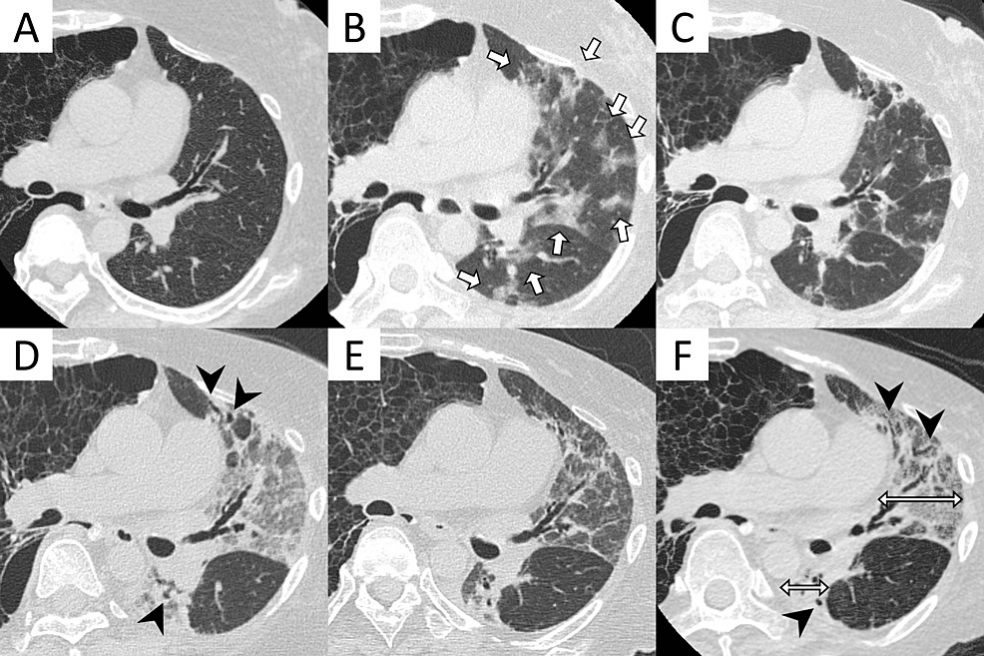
Chest computed tomographies. (A) Baseline CT scan after LTx. The right lung is replaced by cysts due to lymphangioleiomyomatosis, and the left transplanted lung has no radiological abnormality, (B) diagnosis of COVID-19 pneumonia with multiple ground-glass opacities and consolidations (arrows) in the left lung, (C) after treatment for COVID-19, ground-glass opacities and consolidations shrink, (D) with dyspnea progression, broad ground-glass opacities and consolidations appear with traction bronchiectasis (arrowheads) in the left lung, (E) after treatment with a steroid pulse and increased dose of immunosuppressive medication, abnormalities are improved, (F) on the second admission to our hospital - 18 weeks after her COVID-19 diagnosis and 10 weeks after her dyspnea worsened - ground-glass opacities and consolidations with traction bronchiectasis (arrowheads) get worse and the left lung volume decreases (double arrow). This “stair-step” course of lung dysfunction and these typical abnormalities suggest RAS. LTx: lung transplantation; COVID-19: coronavirus disease 2019; RAS: restrictive allograft syndrome
